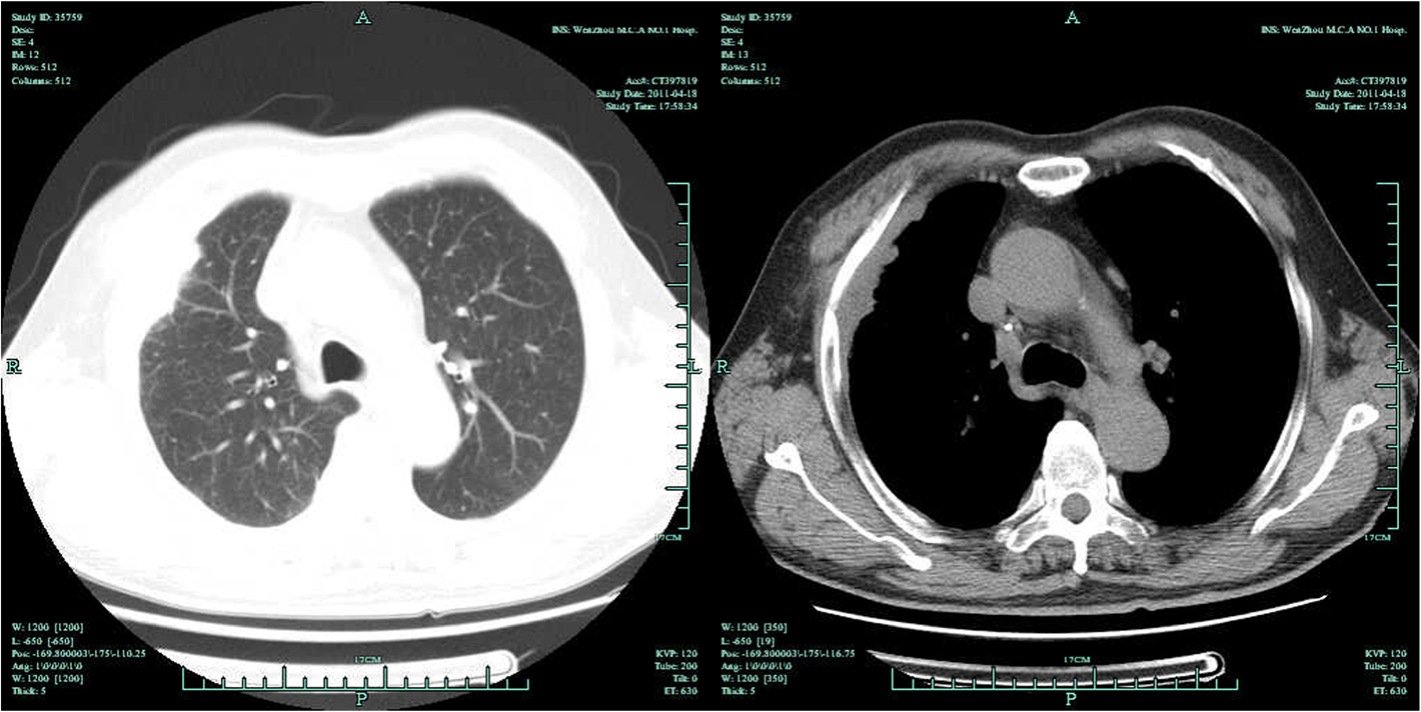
Second chest CT scan on 18 April, 2011.
